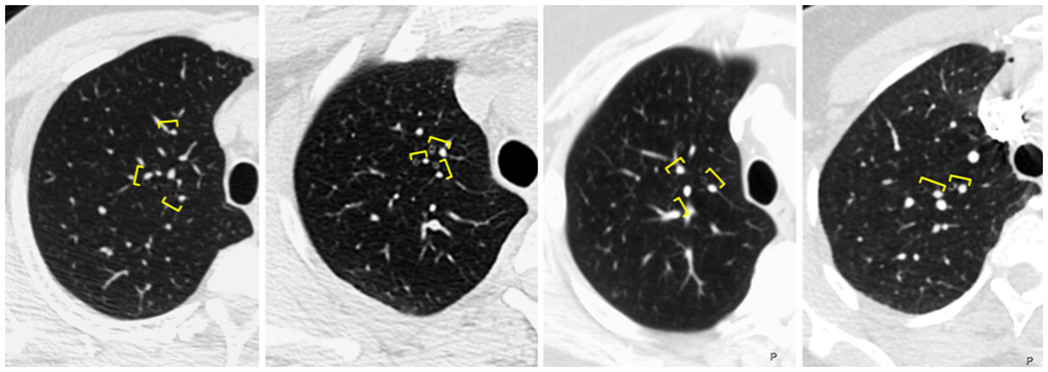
Axial CT images of the right upper zone in a patient with hepatopulmonary syndrome (A), subclinical hepatopulmonary syndrome (B), liver dysfunction only (C), and control subject (D) with representative artery and accompanying bronchus highlighted (brackets).
